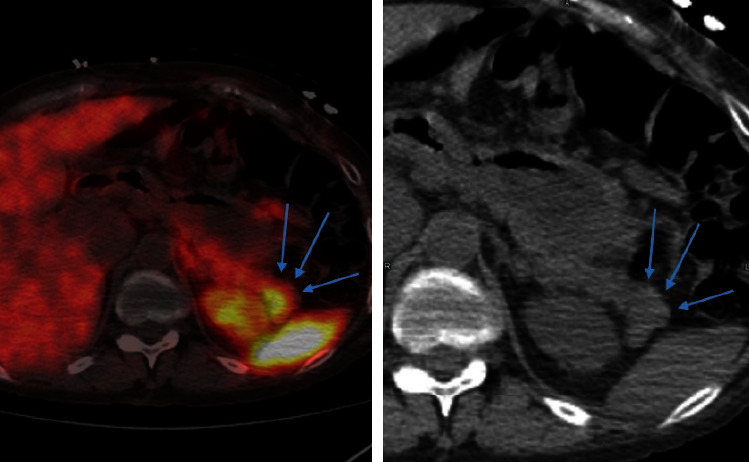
Gallium-68 dotatate PET scan and corresponding abdominal CT. Gallium-68 dotatate PET demonstrates a focal area of radiotracer concentration in the distal pancreas consistent with a neuroendocrine tumor in the tail of the pancreas. Compared to CT imaging of the distal pancreas, there is a contour abnormality near the tail, suggesting a possible lesion.
